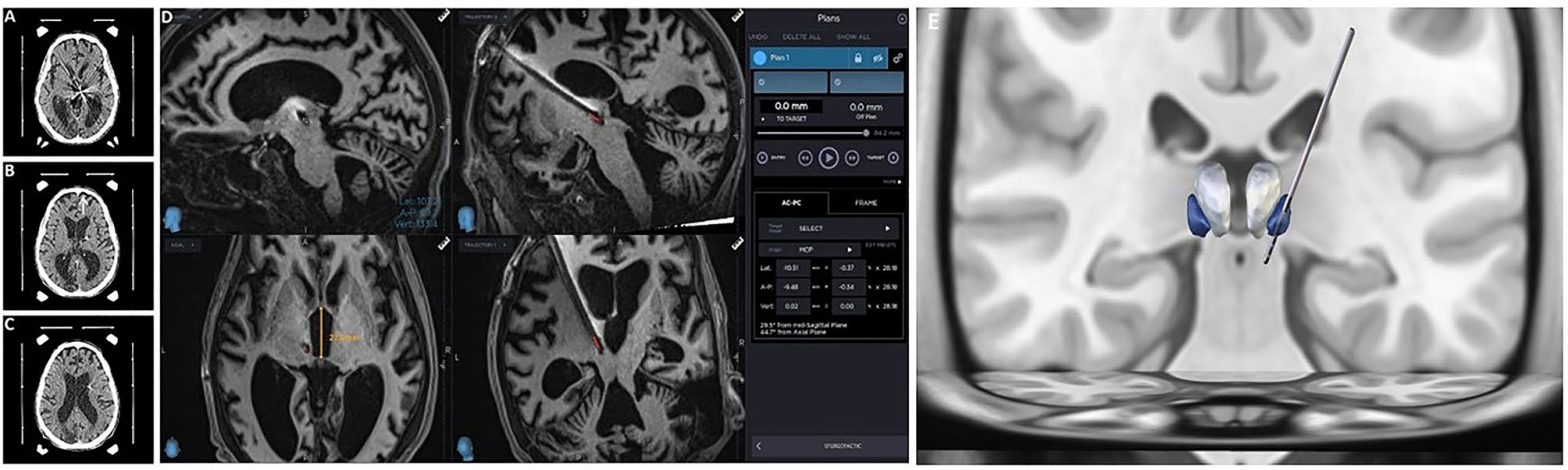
Position of the implanted lead in CM-pf, presented in axial plain on immediate postoperative framebased CT on the level of the thalamus (A), at the level of lateral ventricles with white arrow pointing to intracranial air and absence of brain shift (B), and at the level of lateral ventricles (C). Position of the implanted lead in CM-pf in transversal and sagittal plane presented on early postoperative MRI using Stealth Station (Medtronic), with coordinates on the right side (D). Additionally, reconstructed lead and its position regarding CM (blue) and MD-pf (light grey) nuclei of the thalamus using Lead DBS software (E).
